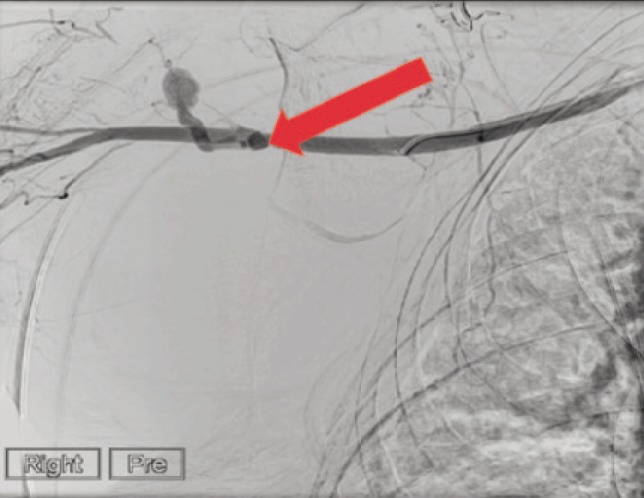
CT Angiogram showing avulsion of posterior circumflex
